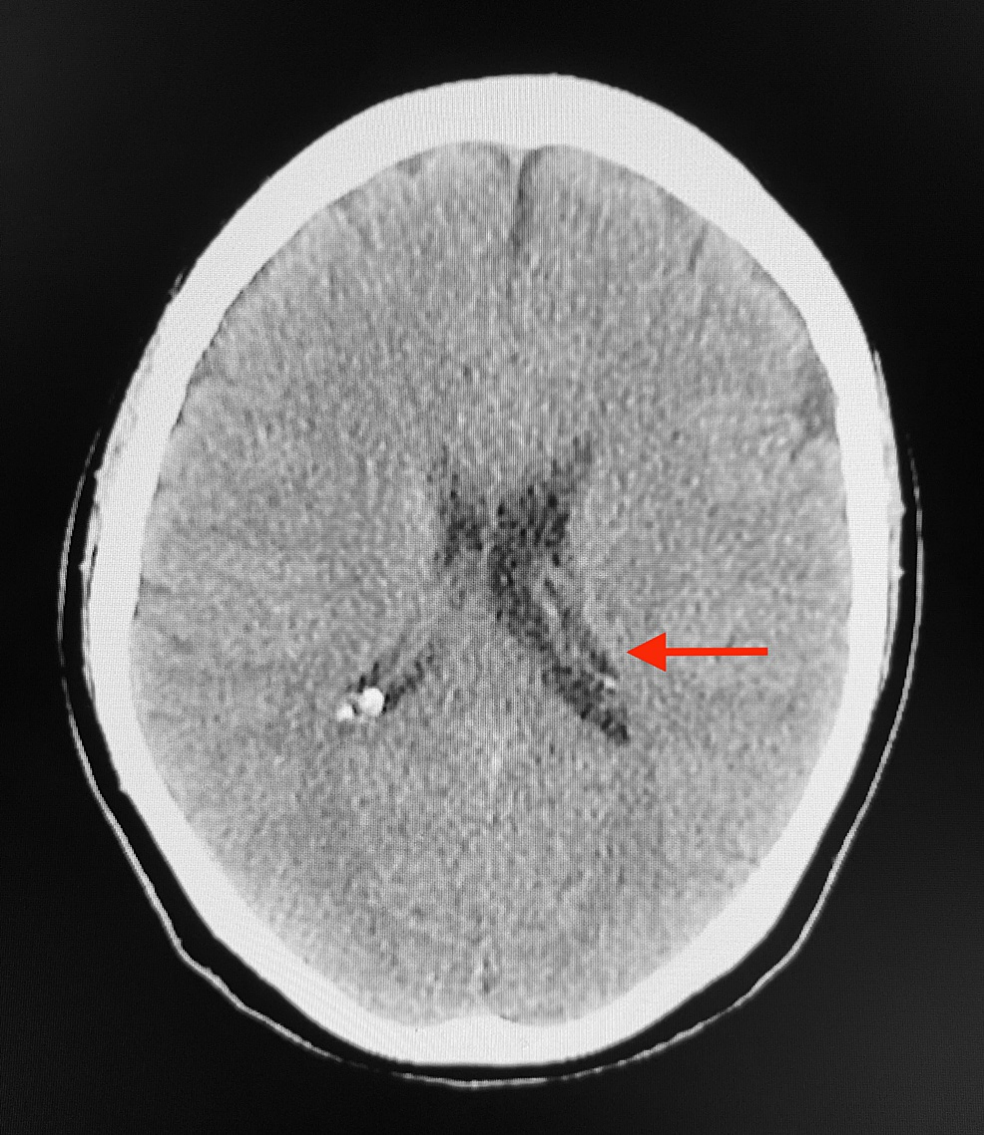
CT of the head without contrast: left lateral ventricle (red arrow) slightly larger than the right, a normal variant No significant intracranial findings.
